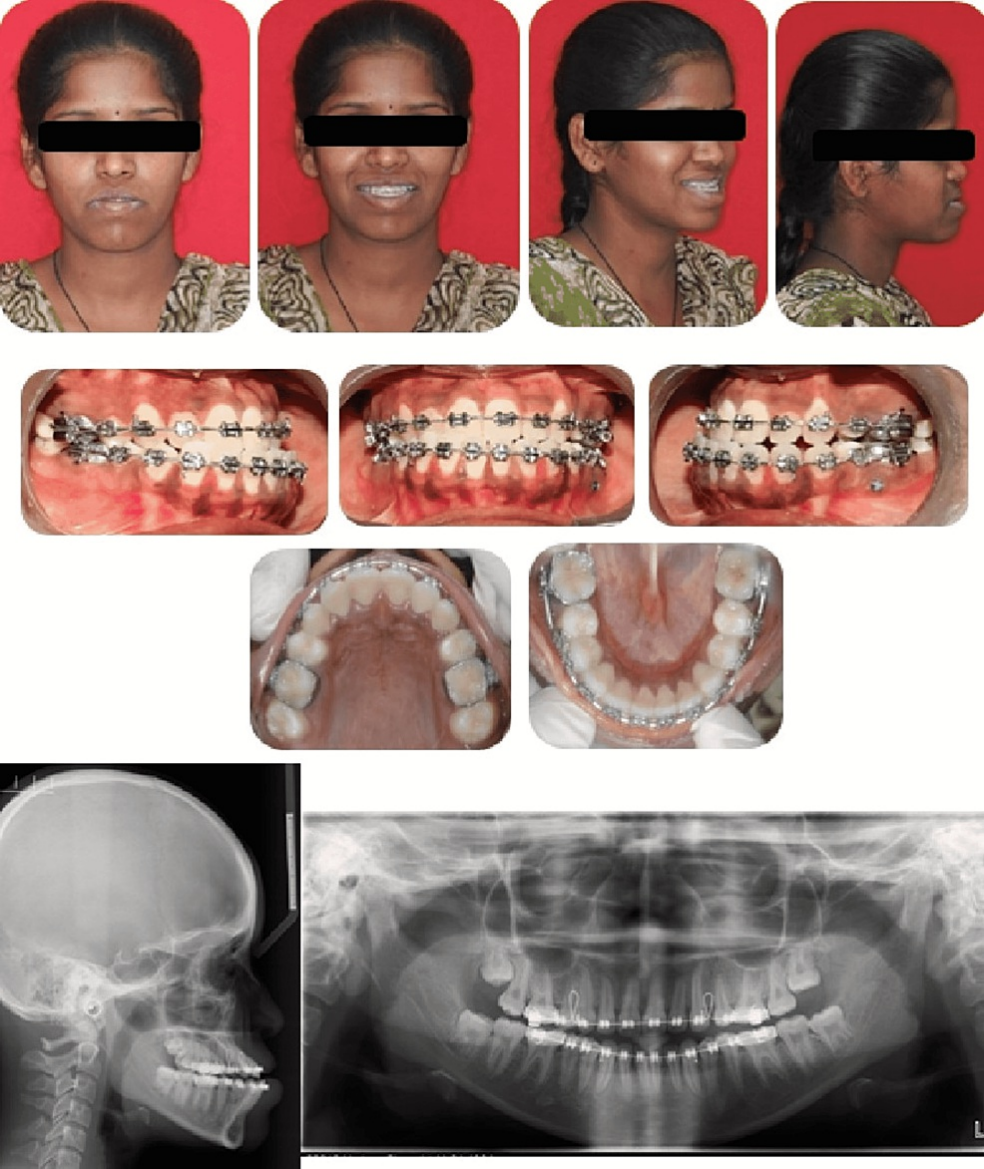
Pre-surgical extraoral and intraoral photographs (lateral cephalogram and orthopantomogram)
